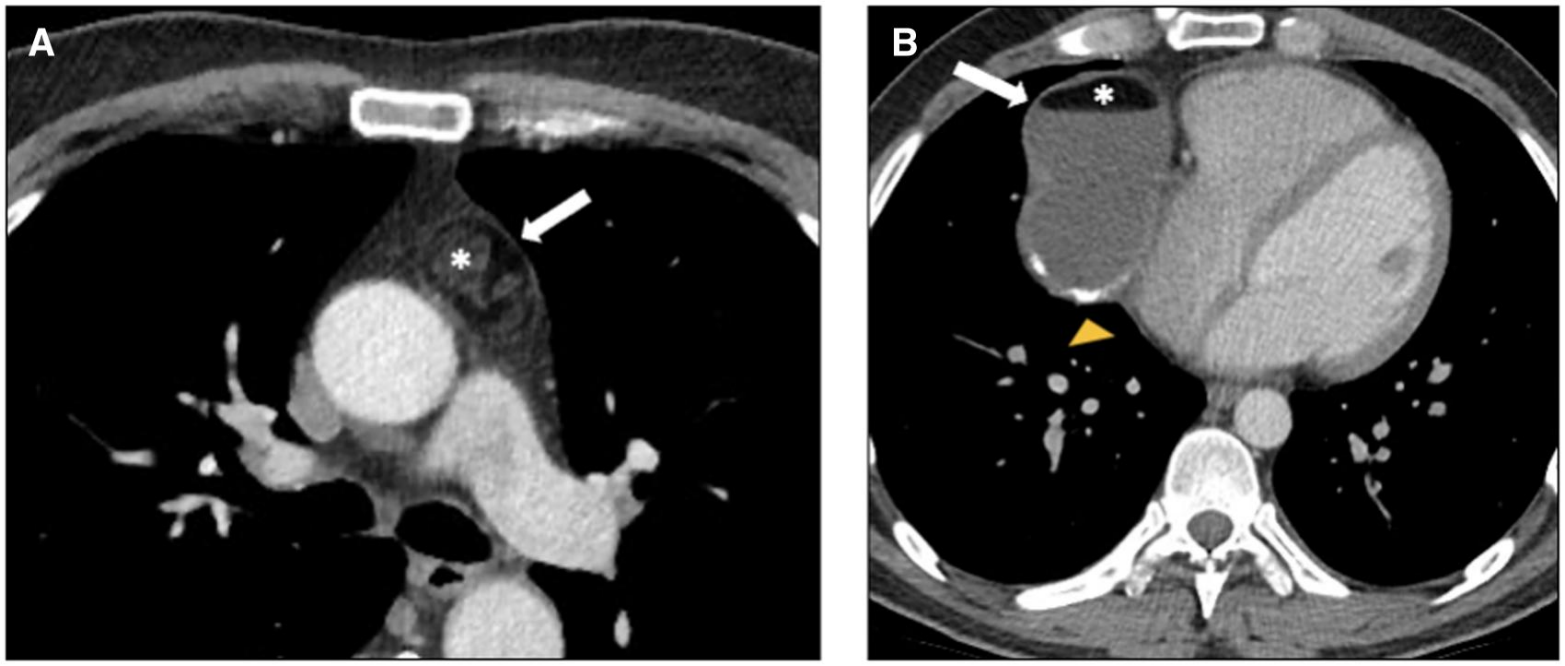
Mature teratoma. (A) Contrast-enhanced axial CT image shows a heterogeneous mass in the left prevascular mediastinum, characterized by encapsulated fatty tissue (arrow) intermixed with soft-tissue components (asterisk). (B) Contrast-enhanced axial CT image shows a right prevascular mediastinal mass with well-defined margins, heterogeneous density, containing both fluid and fat (asterisk) with the characteristic fat-fluid level (solid arrow) and rim-like calcifications (yellow arrowhead).
